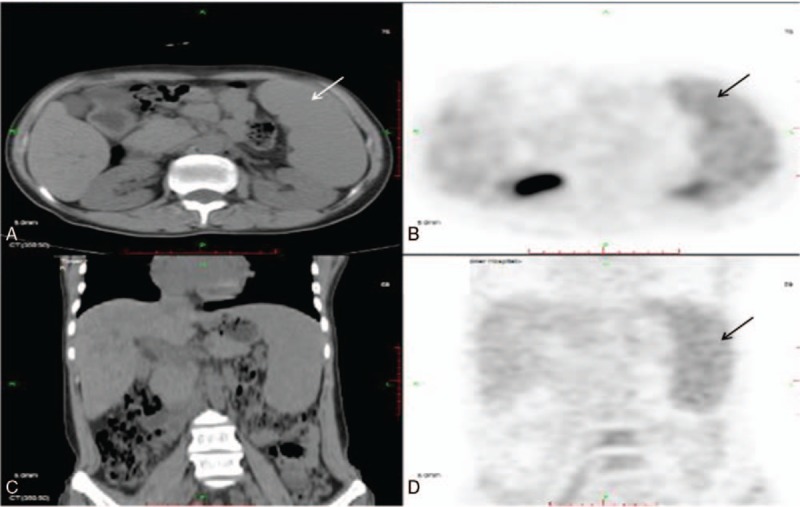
PET-CT on March 27, 2015. (A, B, and D) Splenomegaly and a relatively increased SUV (maximum at 3.3) of the spleen,compared with the liver, was observed. (C and D) No mass or sign of metastasis was observed in the abdominal cavity. The 3 arrows in (A, B, and D) all point at the enlarged spleen.
